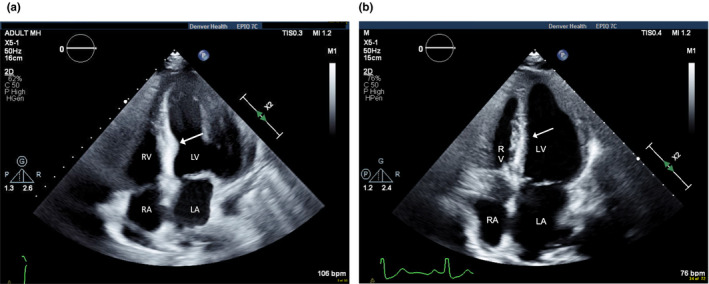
Initial Transthoracic echocardiotraphy (a) demonstrates basal septal bulge (arrow), which resolved (b) after treatment of hypertension. RV = right ventricle, RA = right atrium. LA = left atrium. LV = left ventricle
